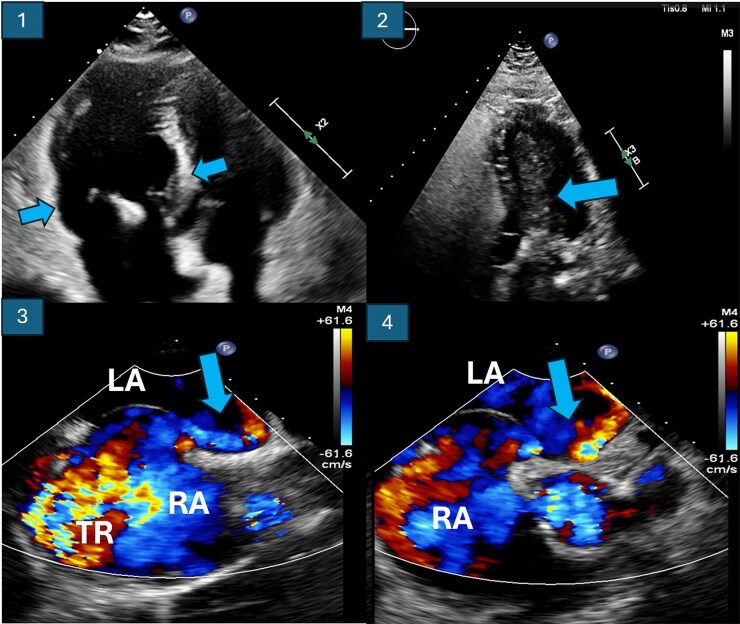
Image 1: Baseline transoesophageal echocardiogram showing right chamber dilation. Image 2: Transthoracic echocardiogram with bubble test demonstrating early massive passage of bubbles into the left chambers. Images 3 and 4: Transoesophageal echocardiogram showing the right-to-left shunt through the patent foramen ovale, as well as the tricuspid regurgitation jet. LA, left atrium; LV, left ventricle; RA, right atrium; RV, right ventricle; TR, tricuspid regurgitation.
